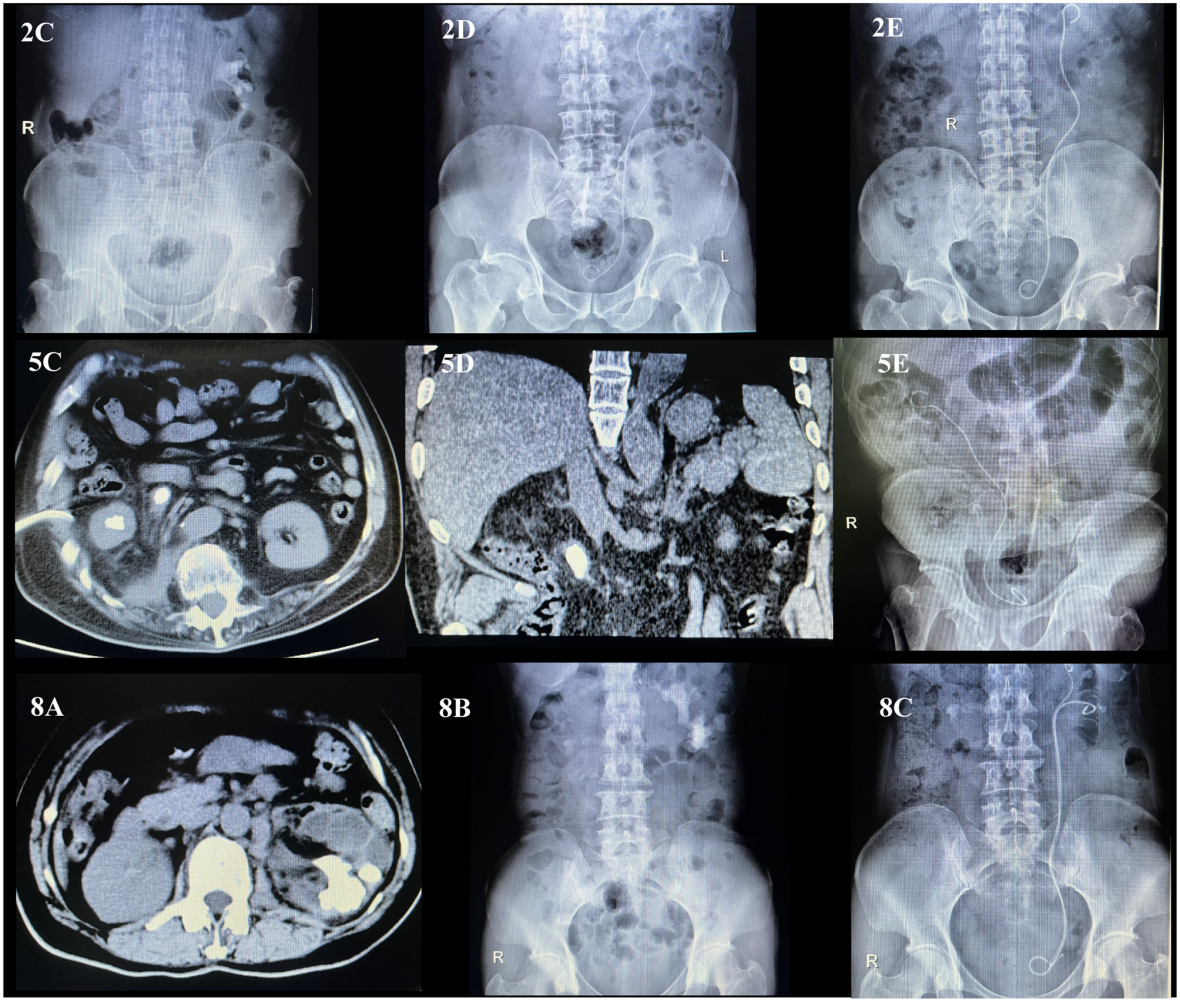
Comparative imaging data before and after operation of patient 2, 5, and 8. Preoperative CT scan results: (2C, 5C, 5D, 8A, 8B). The stone-free rates after twice operation: (2D, 2E). The stone-free rates after primary operation: (5E, 8C).
